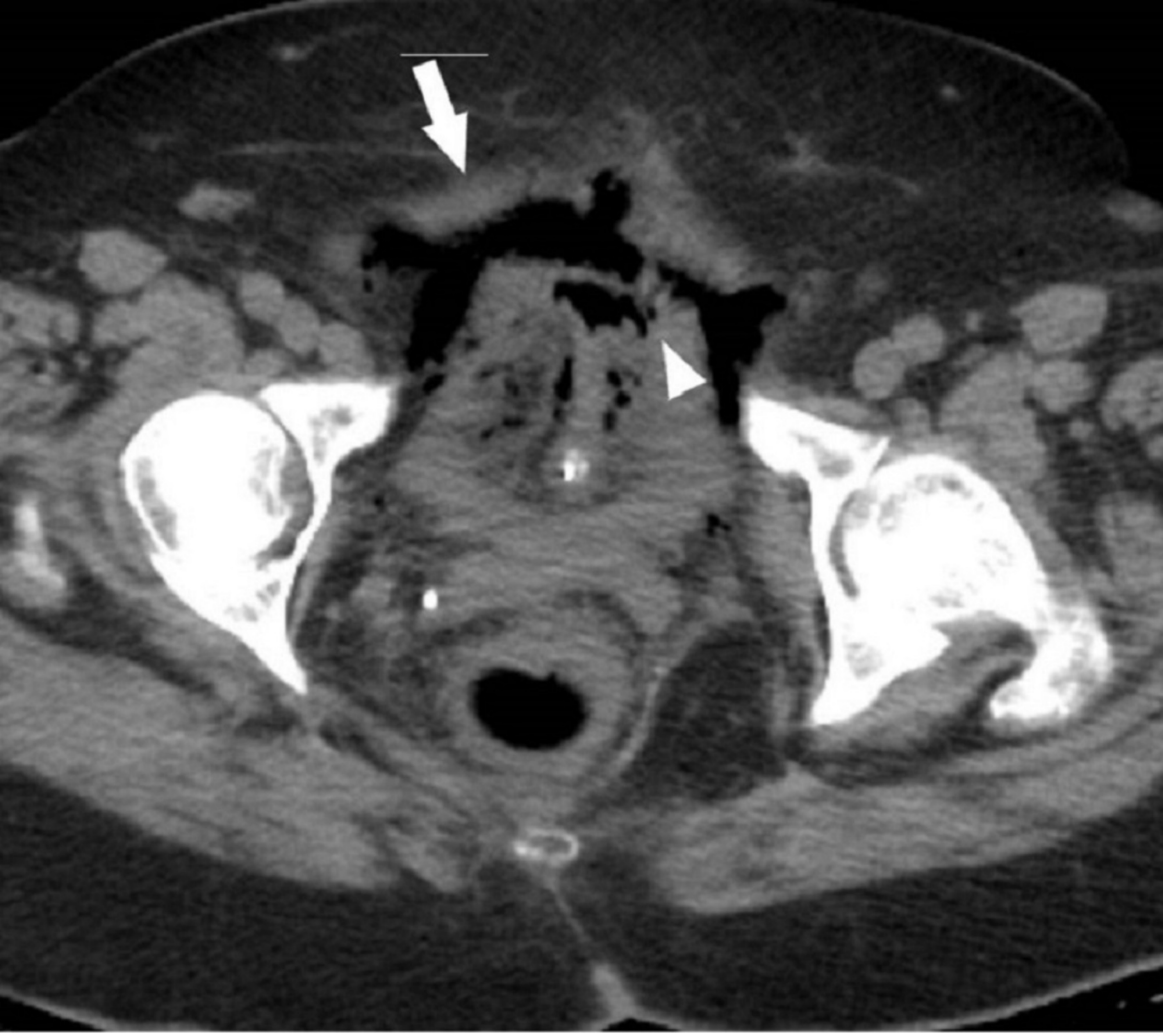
A computed tomographic (CT) scan of the pelvis shows diffuse collection of gas within the bladder wall (arrow head), and air in the peritoneum (arrow)
